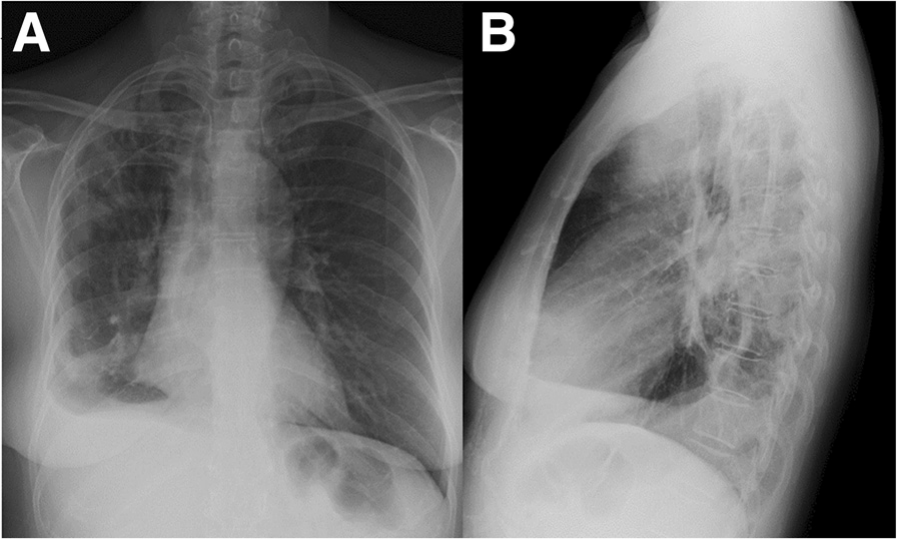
Chest X-ray showing peripheral pulmonary infiltrates predominantly in the right lung with pleural effusion a posterioranterior film, b lateral film
